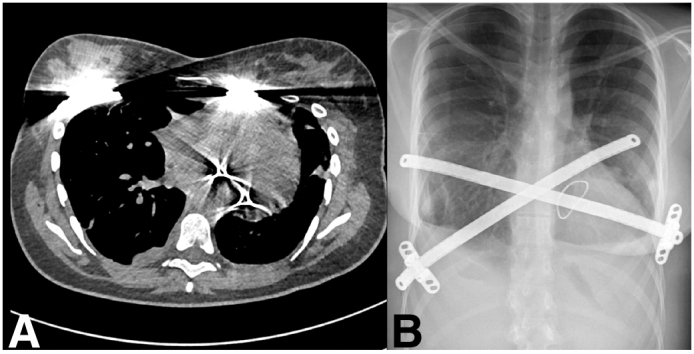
A, Postoperative CT findings: The modified cross-bar Nuss repair in Grand Canyon–type PE showed improvement in the sternal depression (Haller index, 2.06). B, Postoperative chest radiograph after cross-bar technique.
